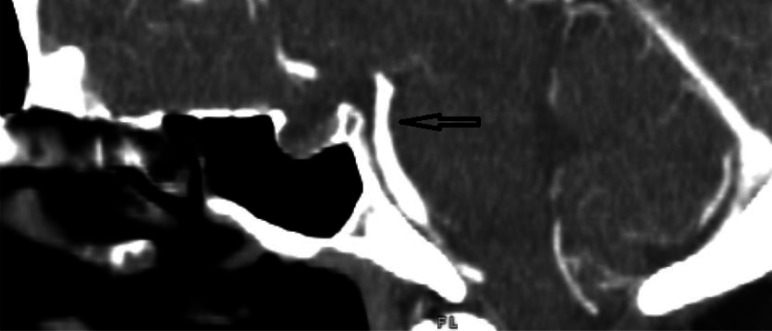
CTA sagittal section 24 days post-diagnosis.
